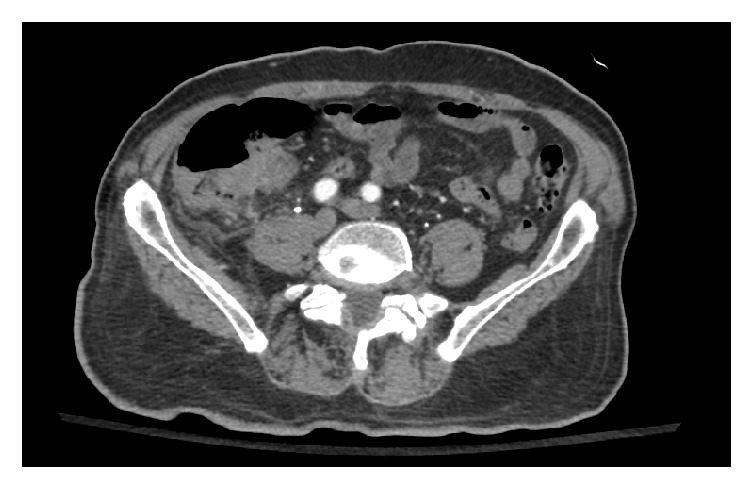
CT abdomen showing evidence of cecal diverticulitis.
